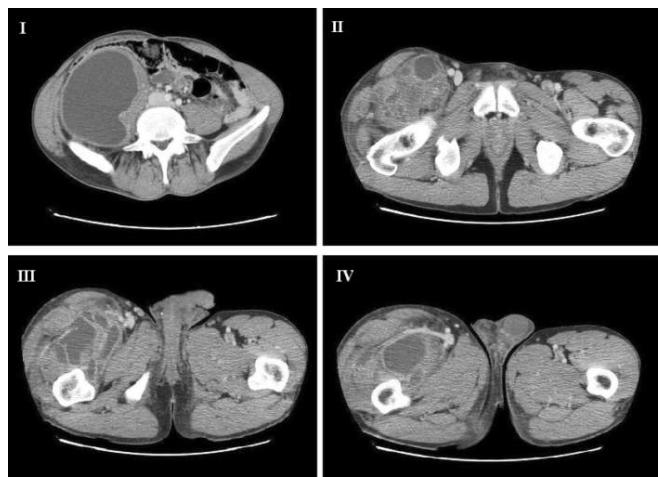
Preoperative basal computed tomography. The involvement of the psoas muscle and retroperitoneum from the Morrison's pouch up to the right iliac fossa and to the iliac region is evident (I, II). The same CT scan shows the involvement of the right thigh with the cysts localized deeply and behind the muscular aponeurotic plane of the femoral quadriceps and abductor muscles up to the knee (III, IV).
